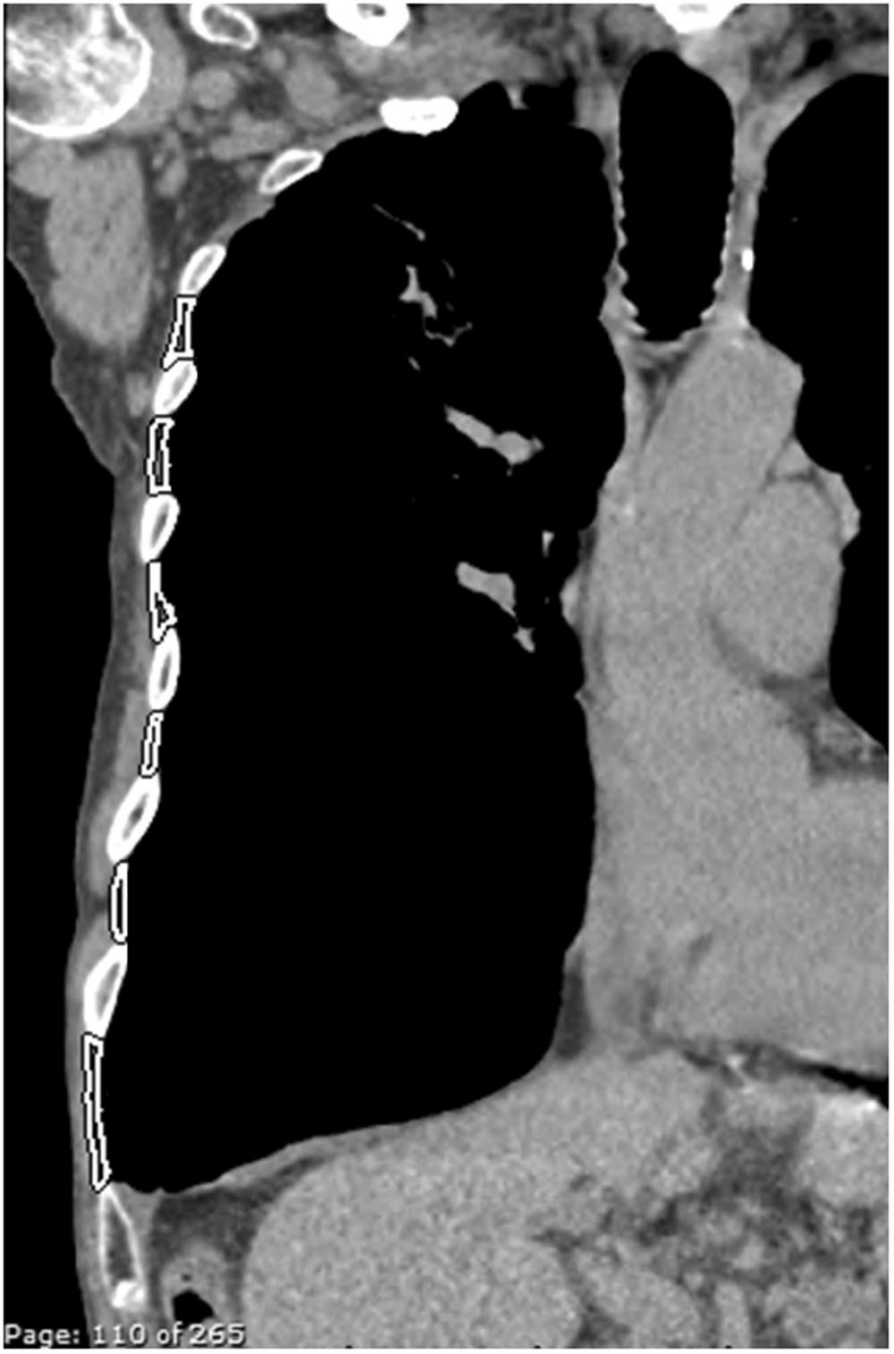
CT image of lateral chest wall internal intercostal muscles. Coronal image from chest CT at lateral arc of 1^st^ rib with ROI surrounding boundaries of 3^rd^–8^th^ internal intercostal muscles.
